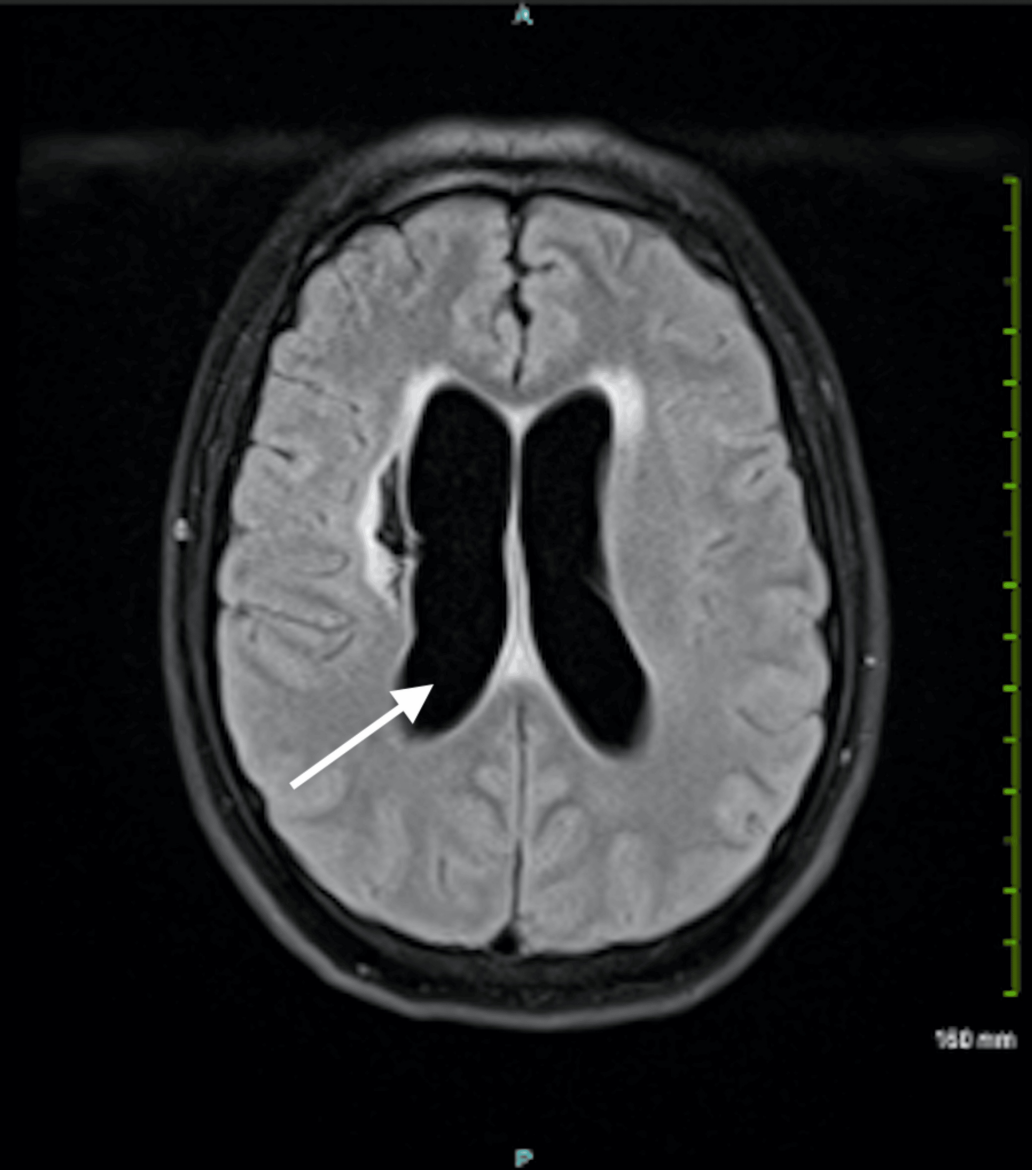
MRI of the head in a patient with coccidioidal meningitis, demonstrating significant ventriculomegaly (arrow) and a complication of hydrocephalus
